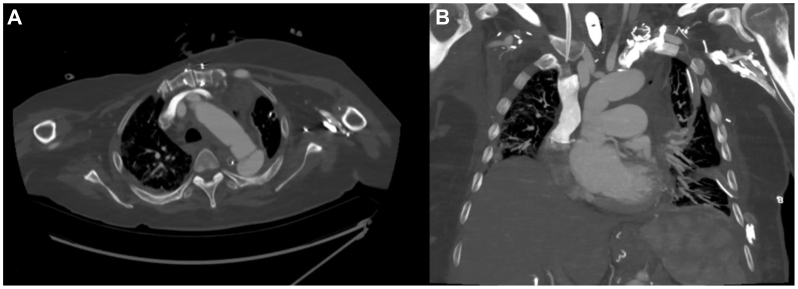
Postrepair computed tomography (CT) scan of the chest after interval resection of the descending aorta and aortic stent with graft replacement. (A) Axial view showing decreased gas foci adjacent to proximal descending aorta with resolution of previously seen gas along distal ascending aorta. (B) Coronal view.
